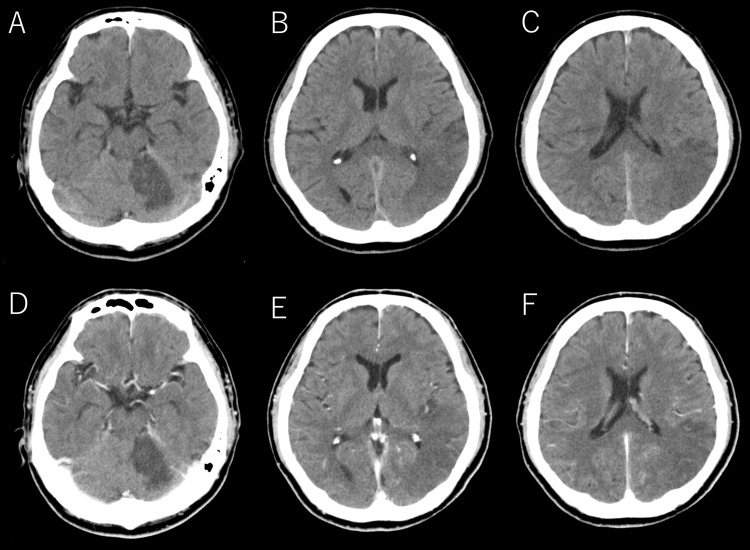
Initial head CT. (A, B, C): Non-contrast head CT images; (D, E, F): Contrast-enhanced head CT images. Multiple infarcts are observed in the left cerebellum, left temporal lobe, and parietal lobe. No evidence of brain tumors, brain abscesses, venous sinus thrombosis, or major cerebral artery occlusion is detected.
